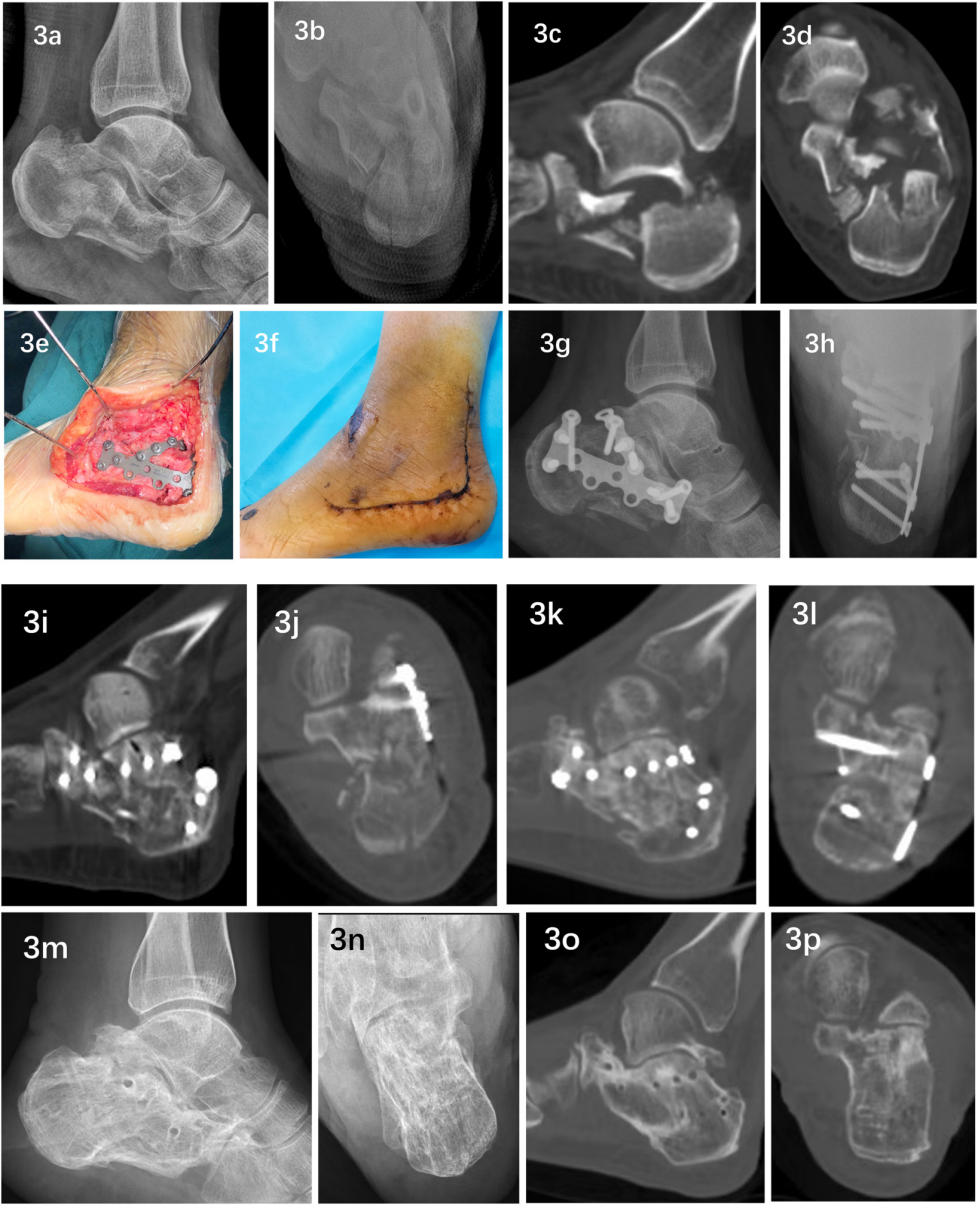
Typical case: A 39-year-old female experienced a closed fracture of the left calcaneus (Sanders type IV) due to a fall from a height combined with a burst fracture of the third lumbar vertebra and no neurological symptoms in either lower limb. Preoperative lateral and axial x-rays (a,b) and sagittal and axial CT scans (c,d) revealed that the calcaneus was highly comminuted and that its shape was basically lost. After admission, emergency open reduction and internal fixation of the third lumbar vertebral body burst fracture were performed. On the 17th day after admission, with the assistance of mirror reconstruction and 3D printing technology (Figures 1, 2), open reduction and internal fixation through the lateral L-shaped expanded approach were performed. During the operation, a precontoured plate and reduced bone were observed. The calcaneus fits well (e) The operation took 55 min, and 45 ml of blood was lost during the operation. The incision healed in the first stage after the operation (f) Postoperative lateral and axial x-rays (g,h) and sagittal and axial CT scans (i,j) revealed that the length, width, height, Böhler angle and Gissane angle of the calcaneus were completely restored, and the posterior subtalar articular surface was completely restored. Collapse and displacement were completely corrected, the articular surface fracture gap was <1 mm, and the varus deformity was completely corrected. The patient was discharged from the hospital on postoperative day 14 and was followed up for 38 months. Twelve weeks after surgery, sagittal and axial CT scans (k,l) revealed complete fracture healing without loss of reduction. Thirty-eight months after the operation, the internal fixator was removed at the patient's request. Lateral and axial x-ray films (m,n) and sagittal and axial CT scans (o,p) revealed no loss of reduction and no obvious calcaneotalar joints. Signs of traumatic arthritis. The AOFAS score at the last follow-up was 96 points, and the VAS score was 0 points.
